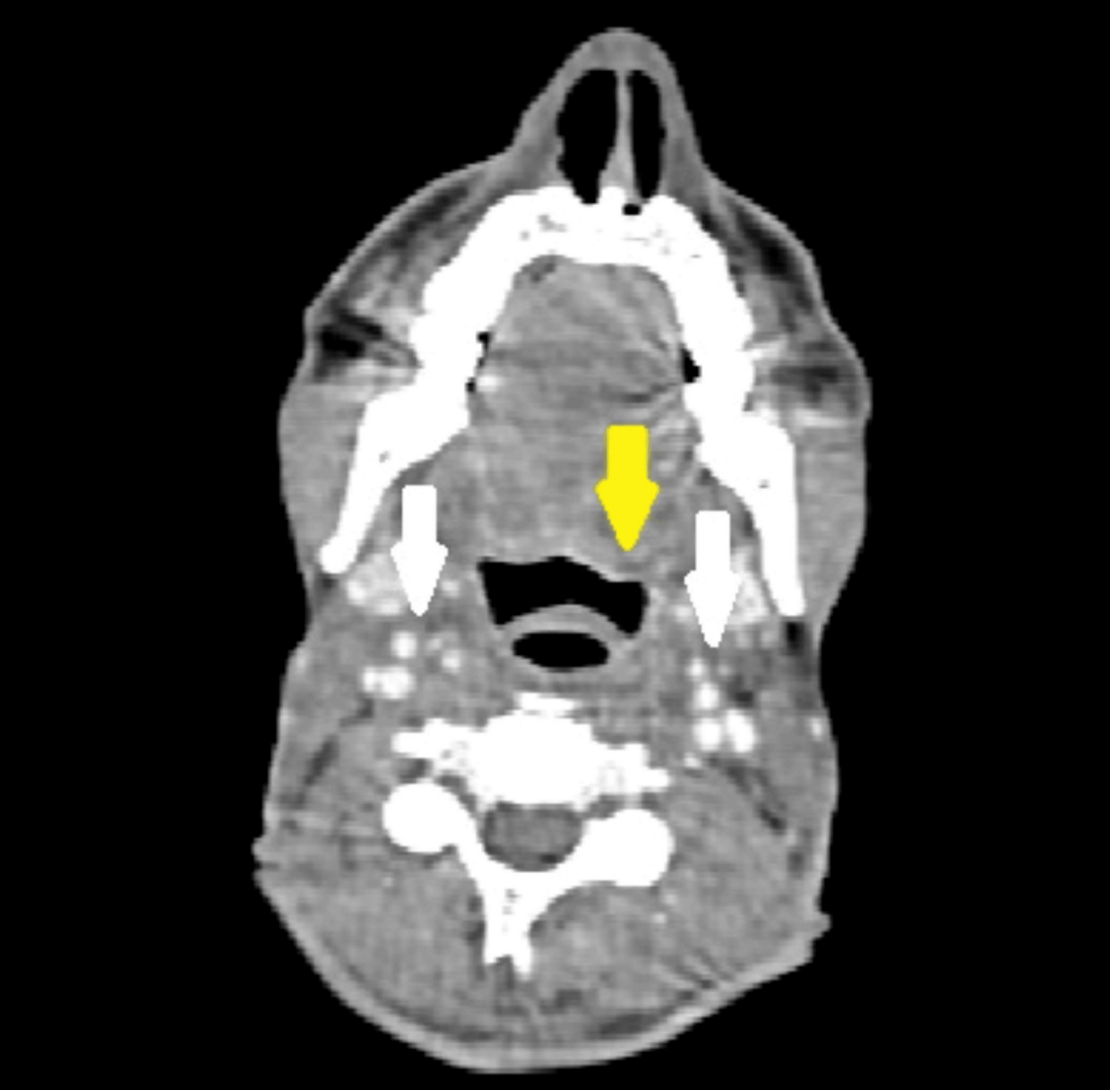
Axial contrast-enhanced CT of the head and neck region. There is no obvious mass or thickening seen in the primary region (yellow arrow) and no bilateral cervical lymphadenopathy (white arrows).
